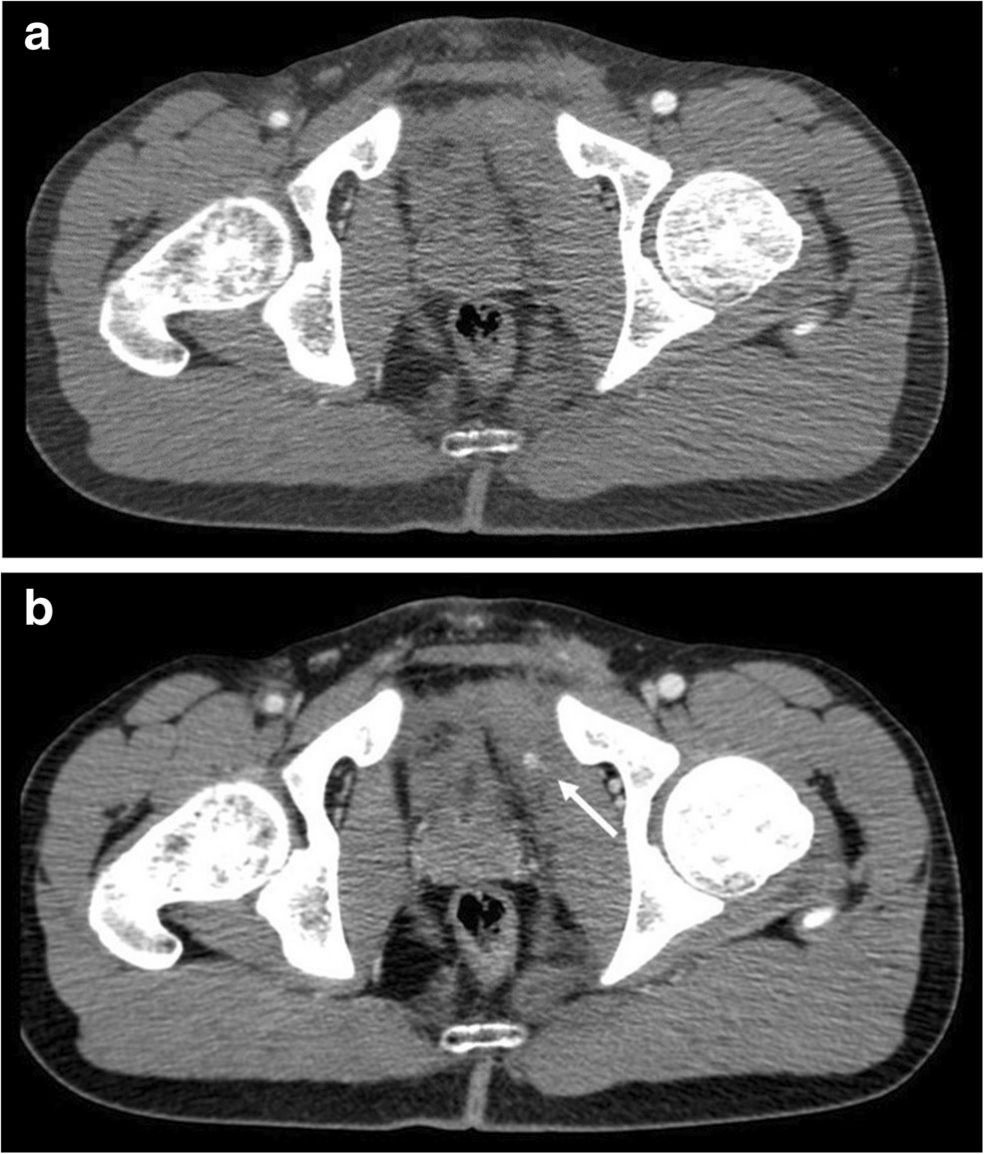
No findings of extravasation in arterial phase CT (a). Extravascular area of hyperattenuation from the left pudendal vascular territory (white arrow) in portal venous phase only (b)
